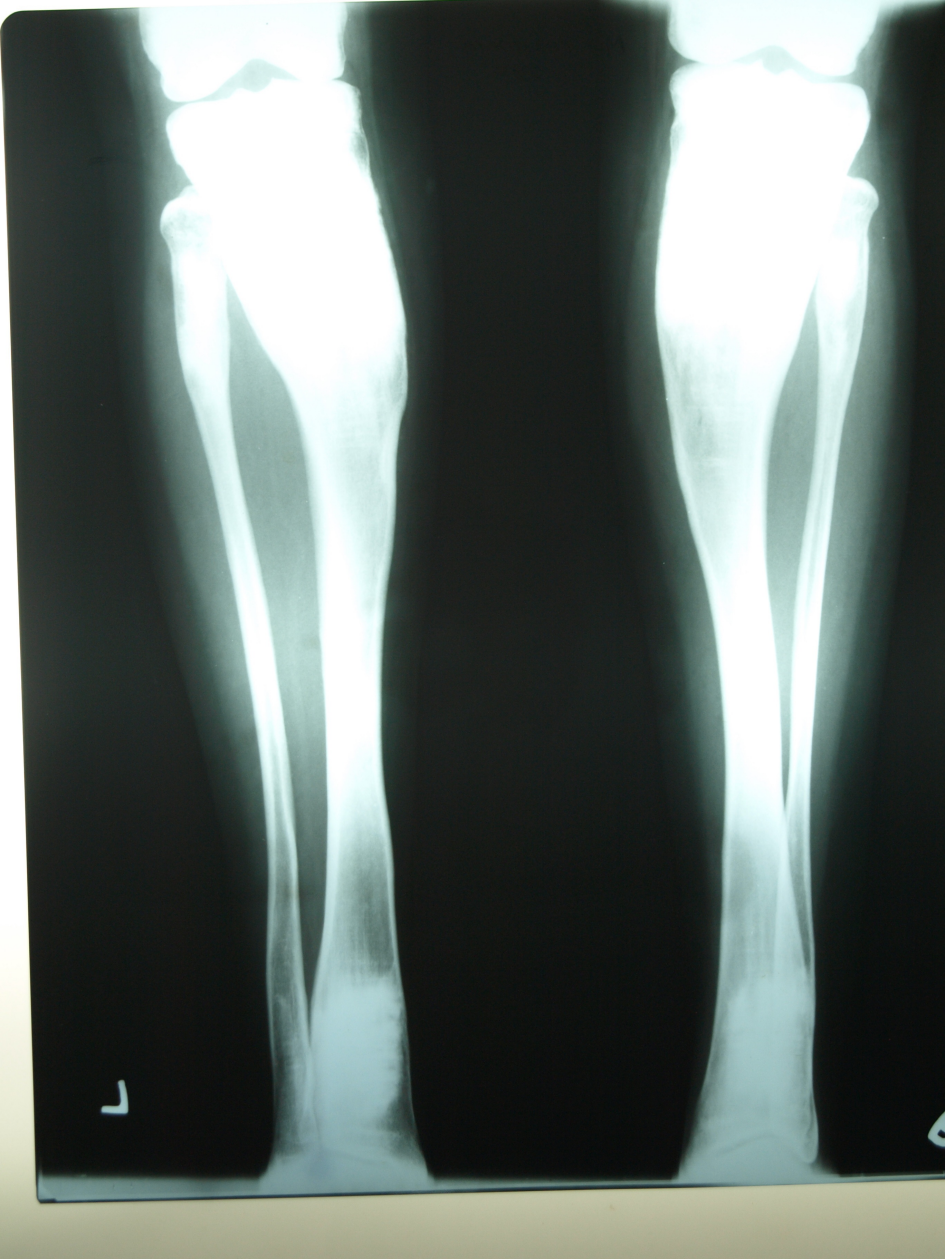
Radiograph of legs showing increased radio opacity
